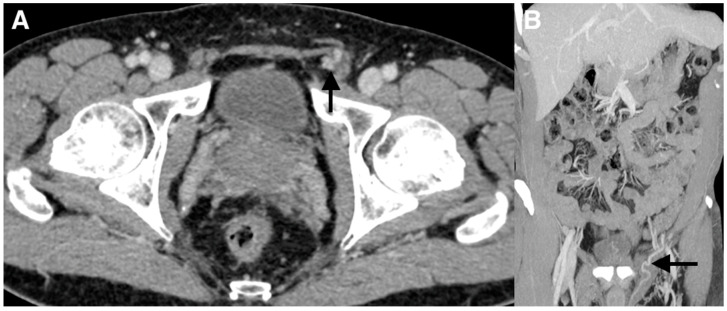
(A,B) Subsequently, a contrast-enhanced computed tomography (CT) imaging study with frontal reconstructions was obtained to evaluate the vasculature. The CT scan did not demonstrate a left testicular vein arising from the left renal vein. The left testicular vein at the level of the inguinal canal was visualized feeding the left-sided varicocele (arrows).
